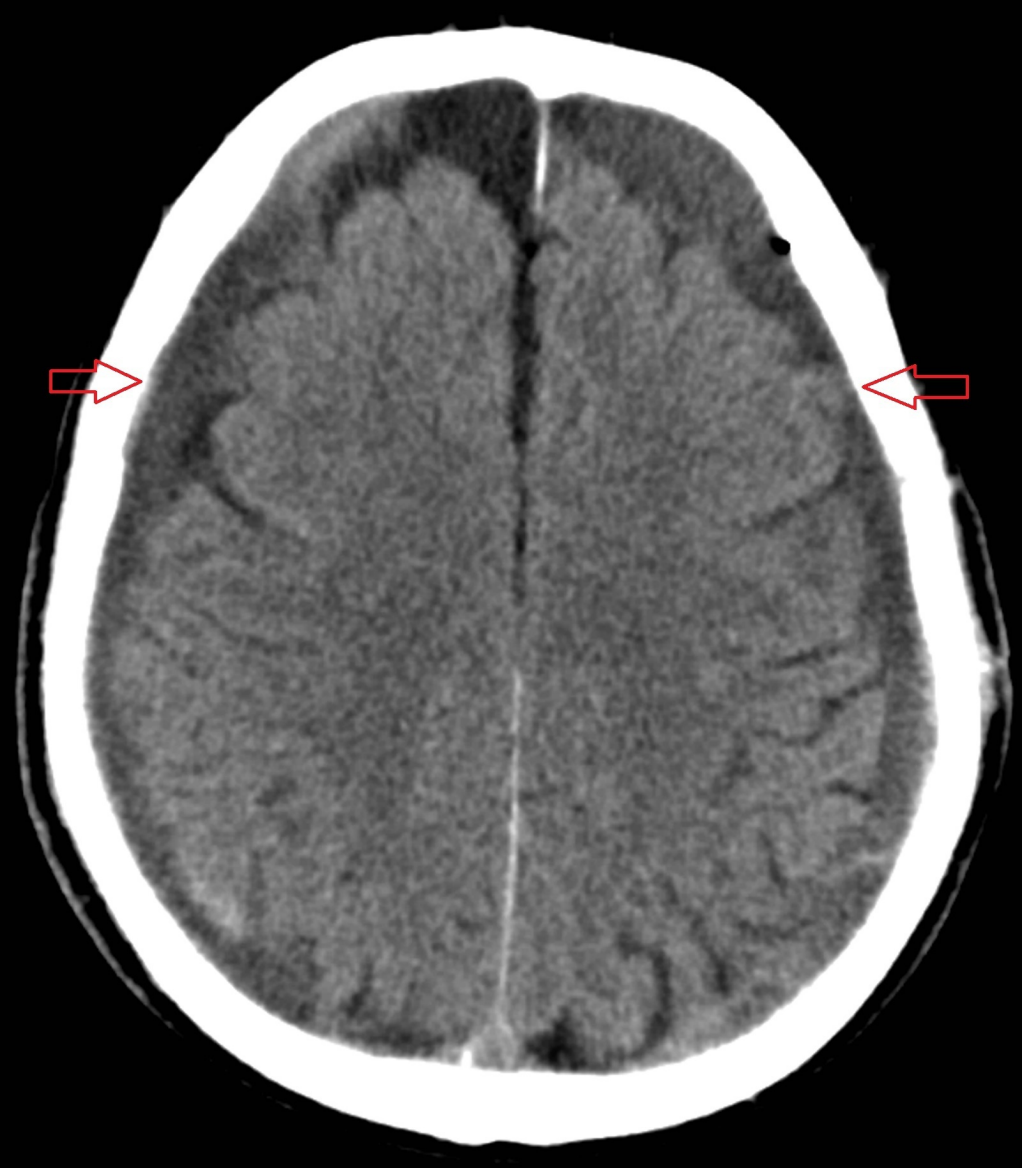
Axial non-contrast computed tomography scan of the brain Demonstrating resolution of the tension pneumocephalus and decompression of the brain.
